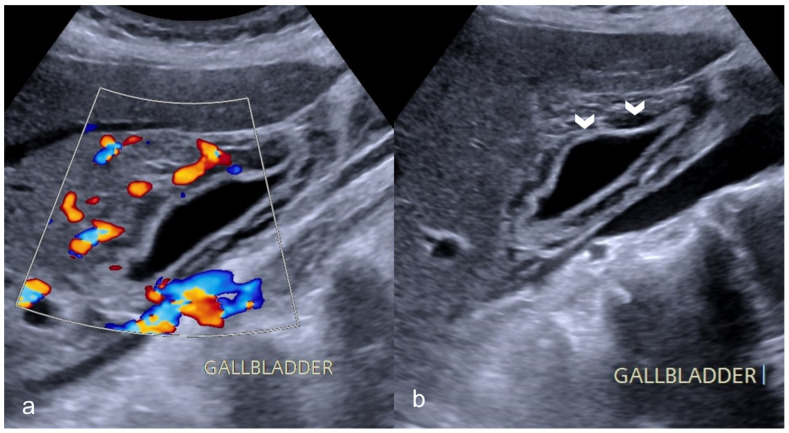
A 69-year-old man with COVID-19 presented a positive Murphy sign. Ultrasound images showed gallbladder wall striation and increased thickness ((b)-arrowheads). The Color Doppler technique revealed hyperemia (a). No gallbladder stones were present indicating acalculous cholecystitis.
